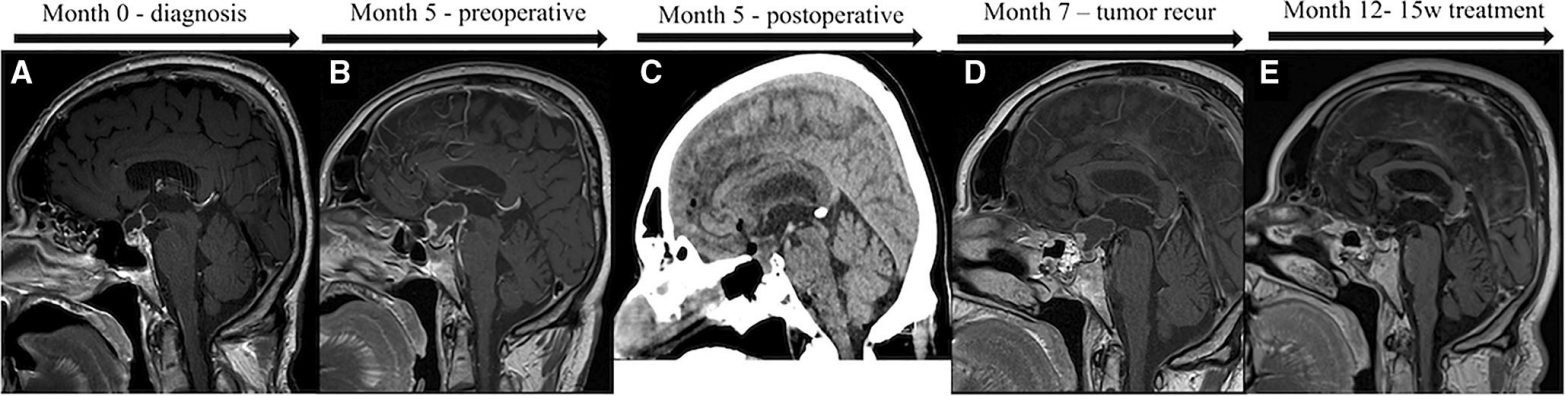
MRI images representing the time of diagnosis (a) until 12 months, which was the last follow-up and 15 weeks following treatment (e). Image b represents the preoperative growth, and image c shows the postoperative CT scan. Image d shows the tumor recurrence/regrowth at 2 months postoperatively
